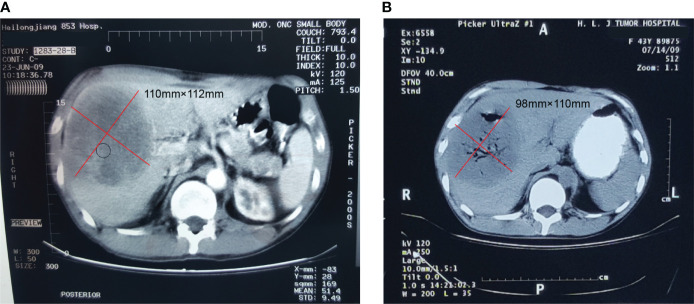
Contrast-enhanced computed tomography of the hepatic extra-gastrointestinal stromal tumor. (A) A 110 × 112 mm mass with solid and cystic components was observed in the right hepatic lobe, with an uneven enhancement in the arterial phase scan. (B) The hepatic mass was treated by argon-helium cryosurgery in July 2009, at which point the tumor was cystic and measured 98 × 110 mm.
